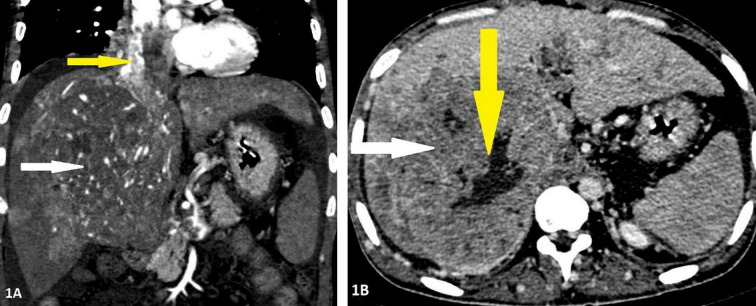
Contrast enhanced computed tomography of 32-years-old male with Hepatocellular carcinoma in the right lobe. Panel 1A: Arterial phase imaging in coronal reconstruction showing the mass having internal areas of arterial hyperenhancement (white arrow) with enhancing tumoral thrombus in the inferior vena cava and right atrium (yellow arrow). Panel 1B: Portal phase imaging in axial section showing washout of contrast in the lesion (white arrow) with central area of nonenhancement suggestive of necrosis (yellow arrow).
